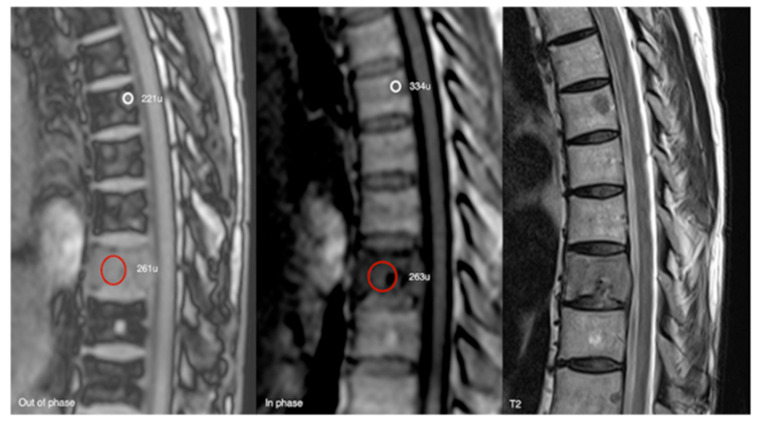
Out-of-phase, in-phase, and T2 sagittal MRI spine as labelled on images (bottom left). Typical haemangioma, upper thoracic spine (221 SI vs. 334 SI), and biopsy-proven breast metastases, lower thoracic region (261 SI vs. 263 SI), with respective values on out-of-phase and in-phase imaging. The calculated signal dropout in the case of the typical haemangioma is 33.8 (>20%) and for breast metastasis it is 0.8 (<20%).
